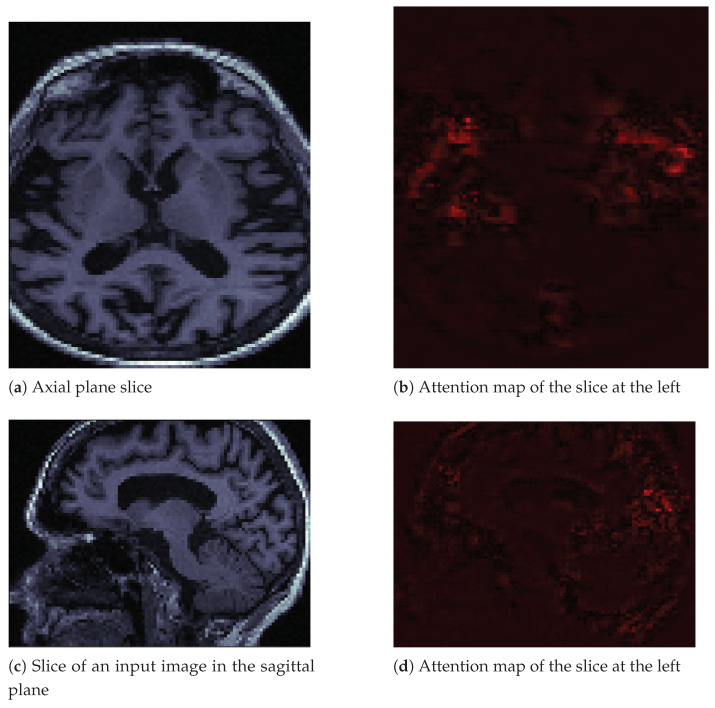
Input image slices and their attention maps from an MRI of a patient with AD.
